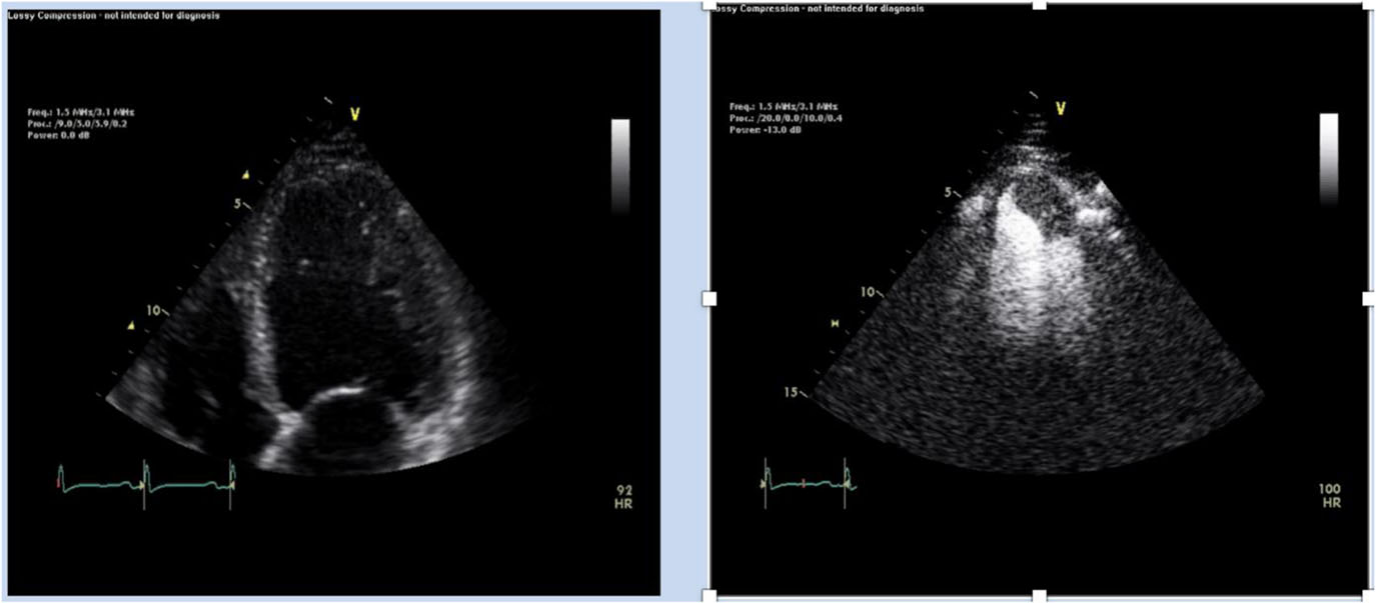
Left Panel: the apical 4 chamber recording before contrast injection while the right panel clearly demonstrates an apical thrombus following administration of an ultrasound enhancing agent
